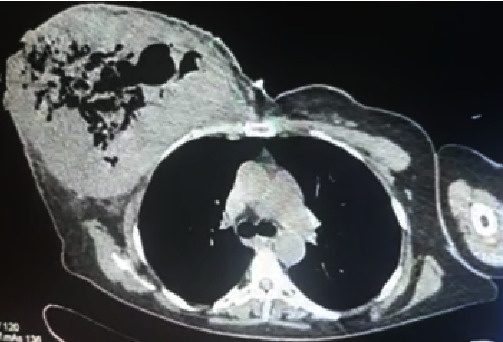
CT scan of the chest showing gas within the right breast and tumor invasion into the right pectoral muscles.
